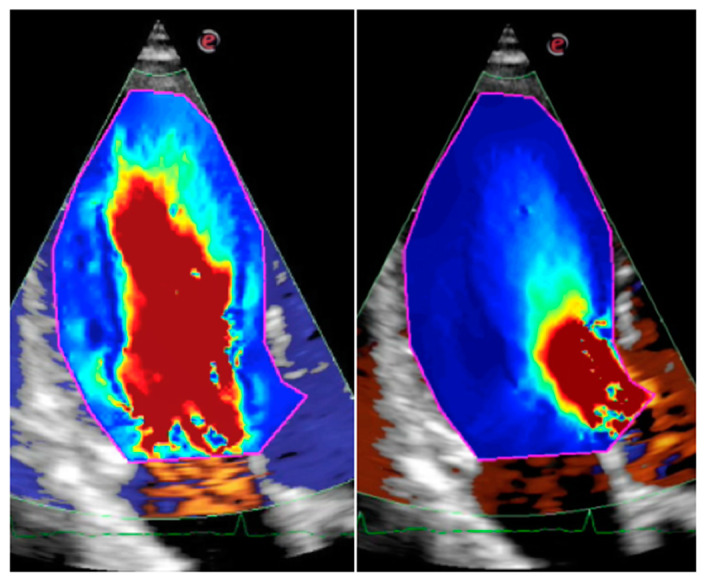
Apical long-axis view of a young normal subject showing the kinetic energy within the left ventricle using the HyperDoppler technique. Blood with the highest kinetic energy is displayed in red color. Left: Early diastole (rapid filling). Right: peak of systolic ejection.
